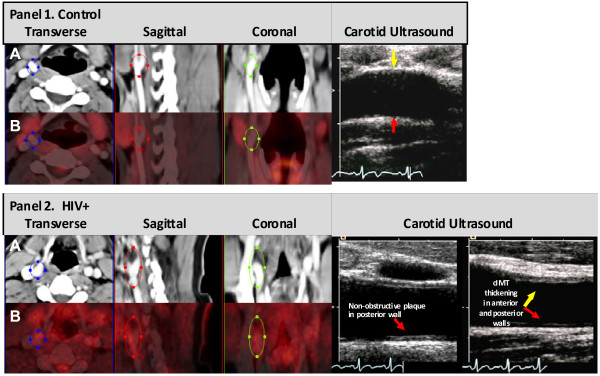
Representative co-registered 3-dimensional positron emission (PET) and contrast-enhanced computed tomography (CT) images of the right carotid artery of a healthy HIV-seronegative control male (Panel 1), and an HIV infected man with CVD risk factors (Panel 2). Transverse, sagittal, and coronal contrast CT images (A) and PET ^18^FDG uptake images (B) along with the corresponding carotid ultrasound images for these two men are shown. The anterior wall of the right carotid artery (upper portion of the carotid ultrasound image) is indicated with a yellow arrow and the posterior wall with a red arrow (lower portion of the image). In the HIV infected man (Panel 2), ultrasound imaging detected increased carotid artery intima media thickness in both the anterior and posterior walls and a non-obstructive plaque in the posterior wall of the right carotid artery. In the healthy control male (Panel 1), the intima media thickness was normal and no plaques were present in the anterior or posterior walls of the right carotid artery. Carotid PET imaging detected regions of higher ^18^FDG uptake (red nodules in blue, red and green ovals) in the HIV infected man (Panel 2B), while less ^18^FDG uptake was detected in the carotid artery of the healthy control male (Panel 1B).
